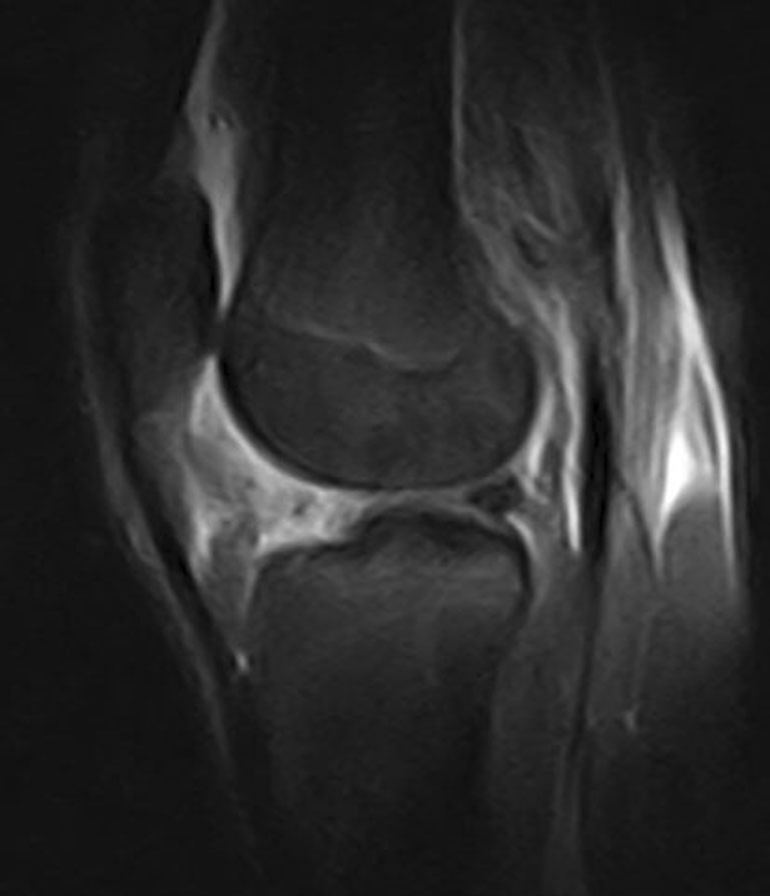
Hoffa’s disease. Repetitive microtraumas with hyperextension and rotational strain in soccer player. MRI proton density with fat saturation images demonstrates an enlarged, oedematous HFP
